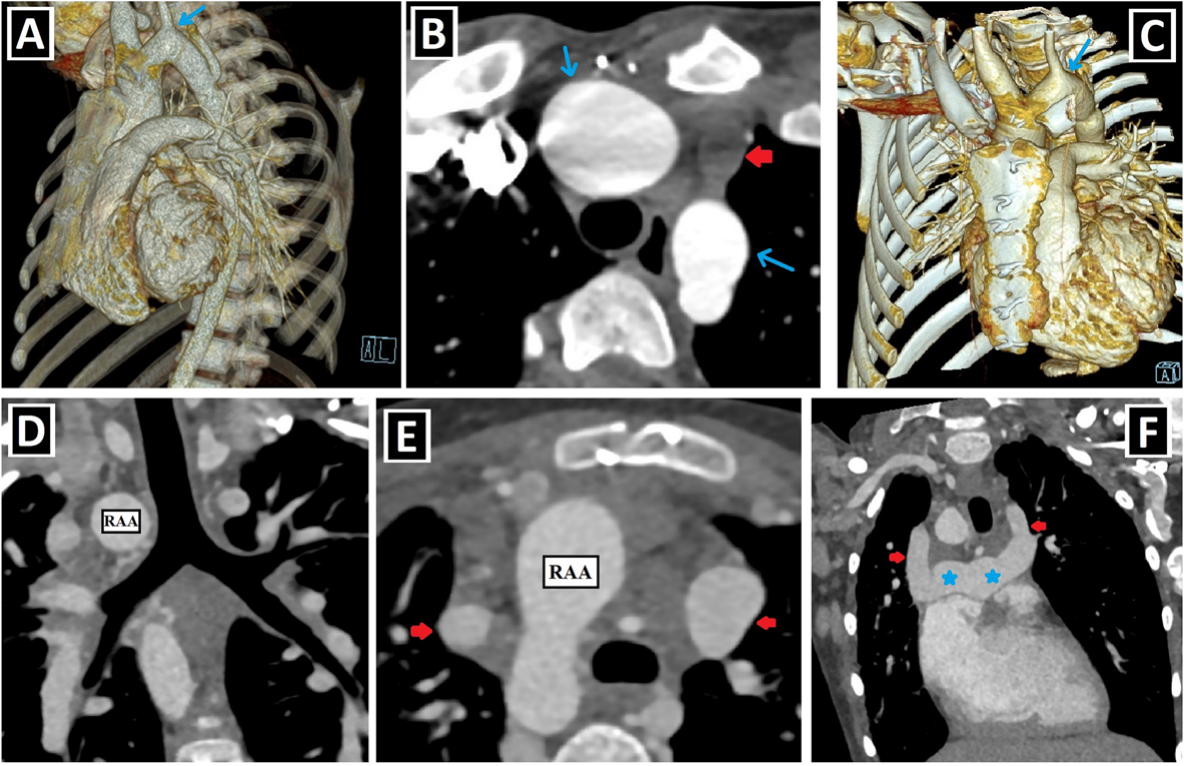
Aortic arch anomalies accompanying PLSVC-2. a–c 3D VRT images with different views (a, c), and axial CT image (b) indicate the cervical aortic arch (blue arrows) in a patient with PLSVC (red arrows). d–f Coronal-oblique reformatted (d, f) and axial (e) CT images depict right aortic arch (RAA) and bicaval Glenn shunts (red arrows) in a patient with double SVC and complex cardiac anomaly. Blue stars represent bilateral pulmonary arteries
